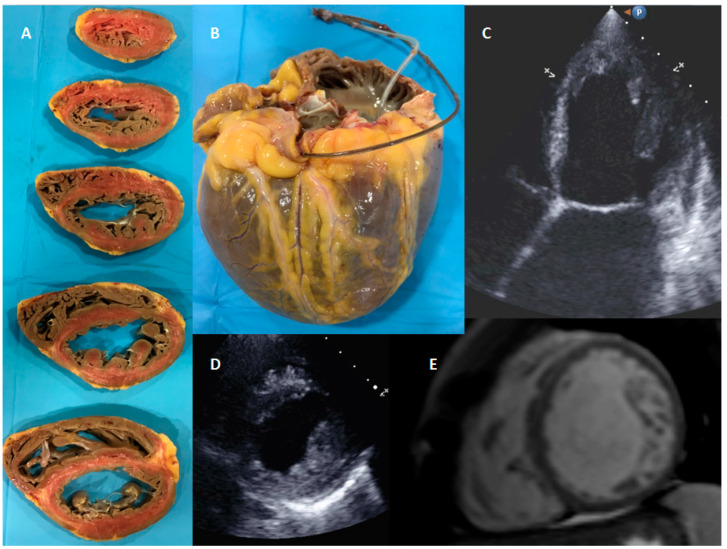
Transplanted patient with isolated left ventricle non-compaction cardiomyopathy diagnosed by CMRI and confirmed by anatomopathological examination. Panel (A) Transversal slides of the explanted heart. (B) Spheroidal shape of the transplanted heart. (C) Apical 4 chamber view in TTE. (D) Parasternal short axis in TTE. (E) LVNC in CMRI.
